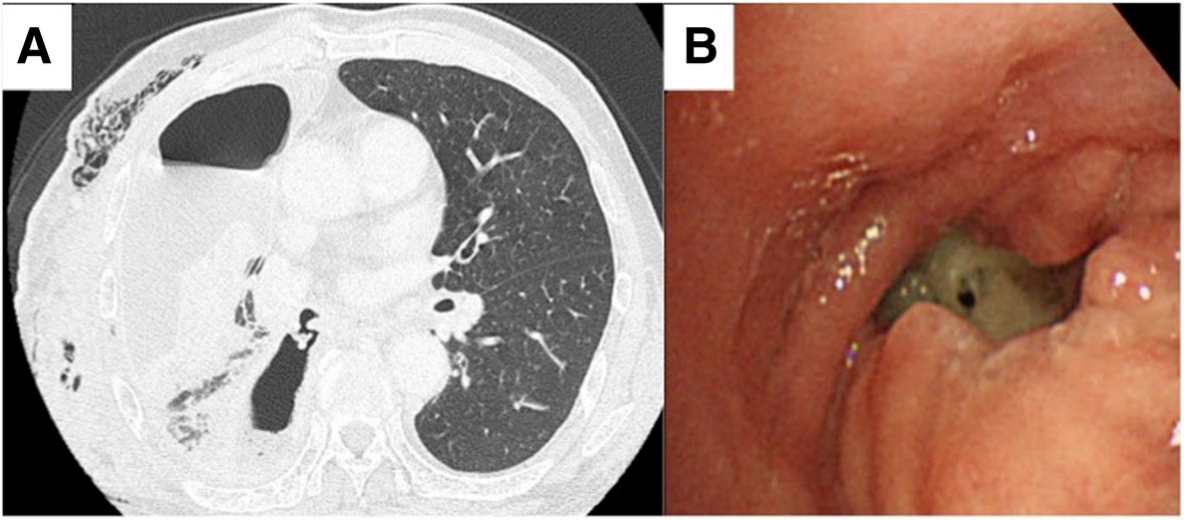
Detection of the bronchopleural fistula (BPF) via bronchoscopy and computed tomography (CT). a Chest CT showed the BPF but no aspiration pneumonia. b Bronchoscopy showed the BPF at the right lower bronchial stump
